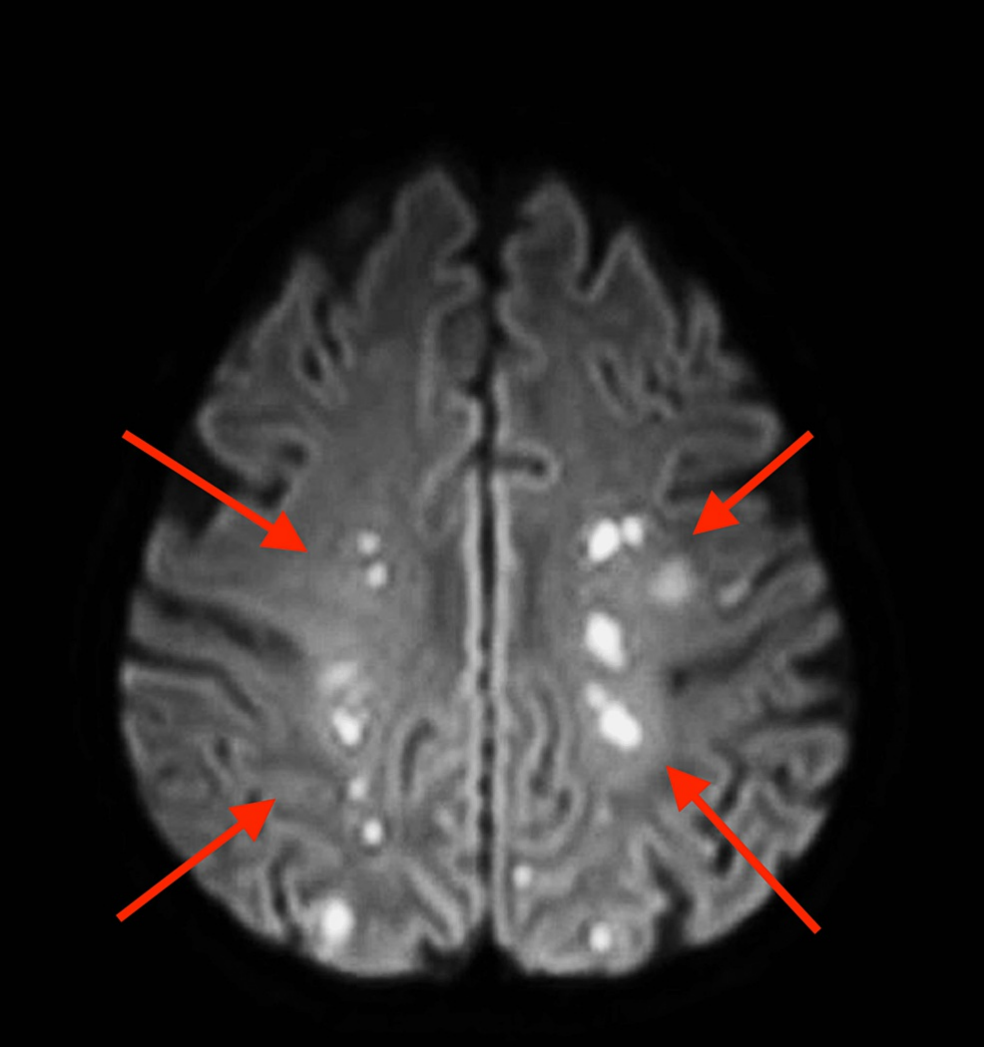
Brain MRI scan showing multiple ischemic lesions Red arrows point to evident ischemic areas spreading in both cerebral hemispheres.
